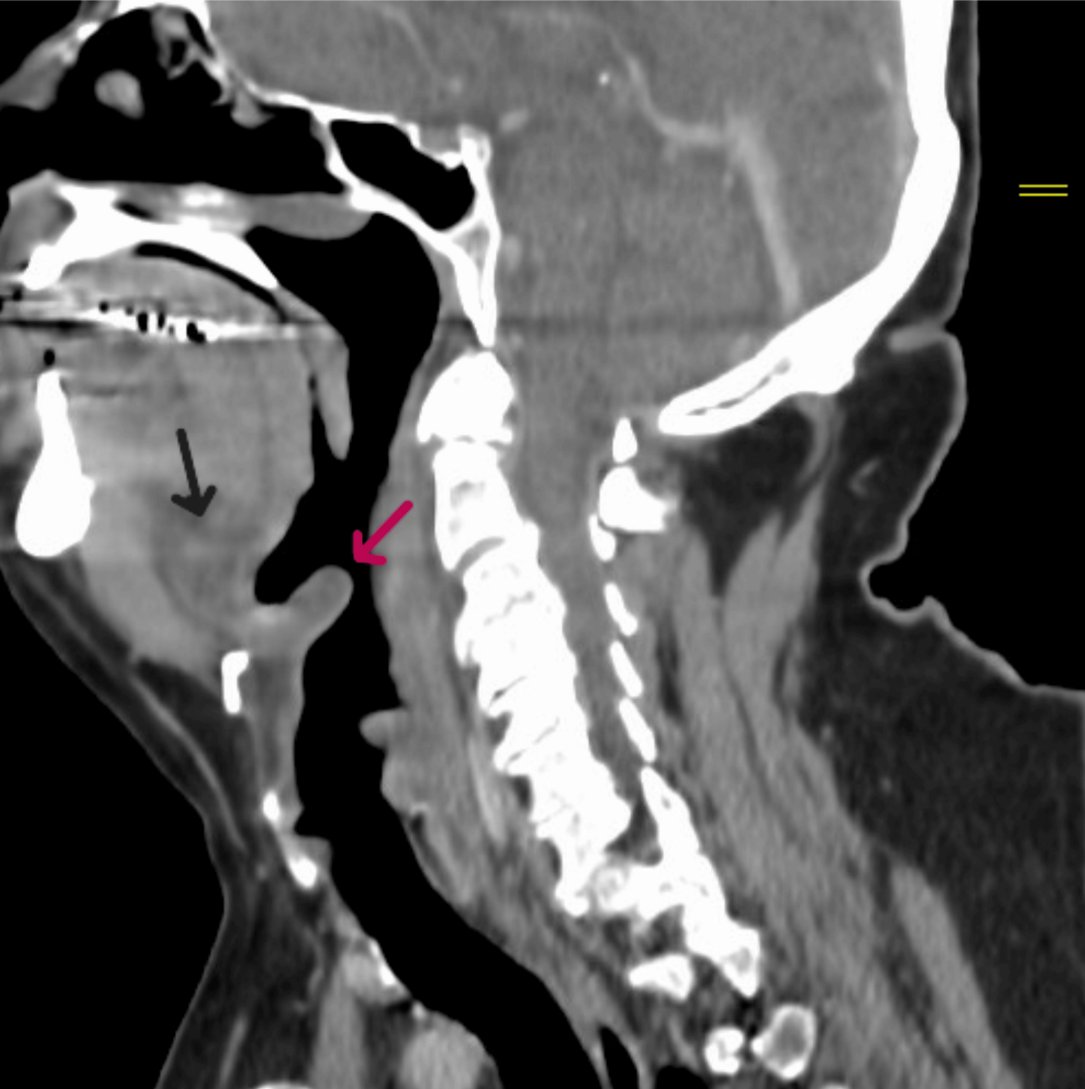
CT image showing edematous epiglottis (red arrow) along with edema of the floor of the mouth (black arrow) CT: computed tomography
